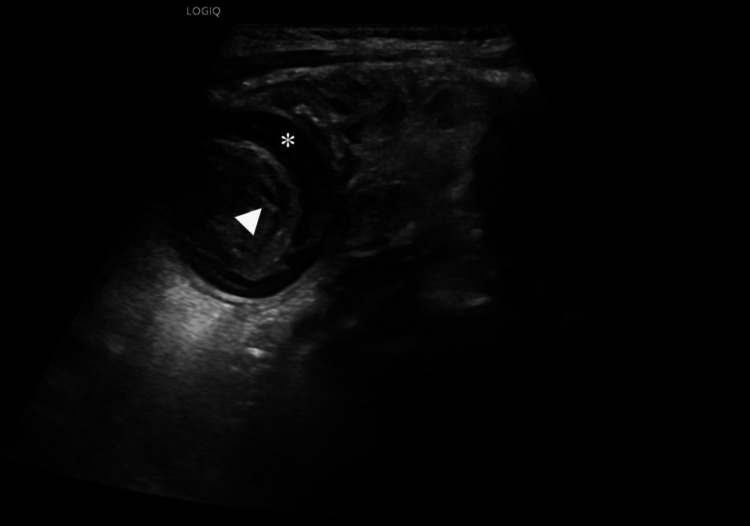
Gray-scale ultrasound image indicating ileocolic intussusception Gray-scale transverse plane reveals a target-like lesion with a hypoechoic rim (asterisk) and hyperechogenic concentric rings (arrowhead), resembling the classic “donut sign”
